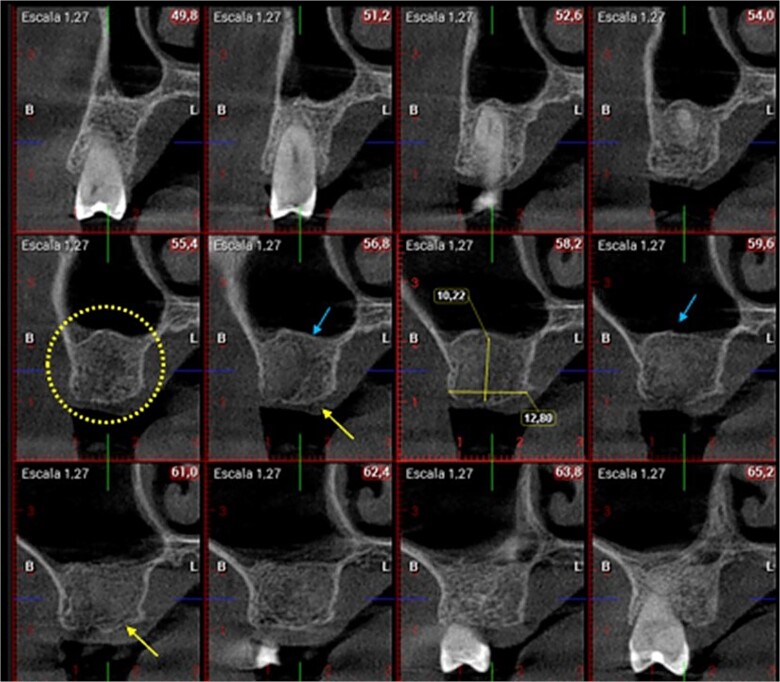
CBCT view 12 months after surgery.
